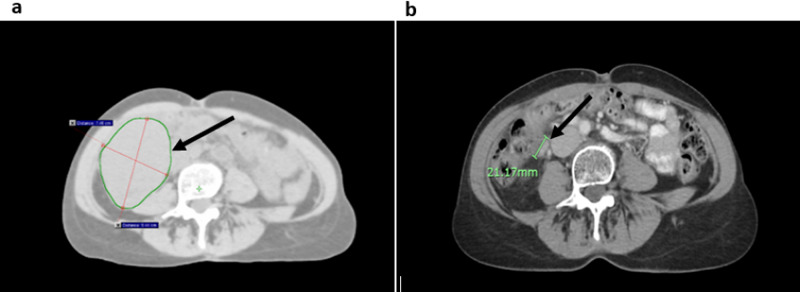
Recurrent right upper quadrant GCT before and after radiation therapy Axial CT abdomen and pelvis obtained (a) prior to radiation, demonstrating a large right upper quadrant mass outlined in green, and (b) three months after completing radiation, where the maximum tumor diameter is demarcated in green. GCT: Granulosa cell tumor
